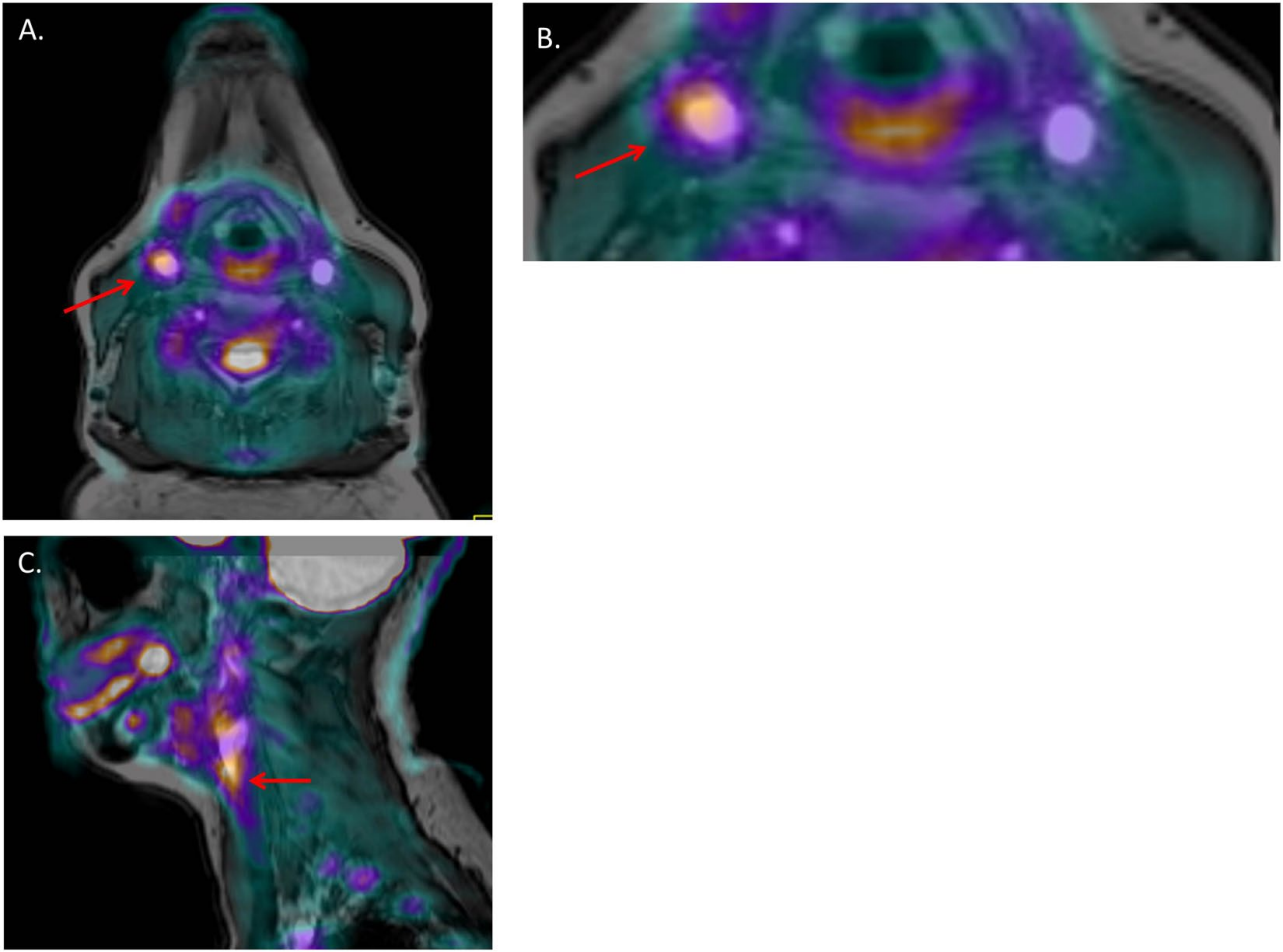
Provides an example of a carotid FDG-PET-MRI in an RA patient. (A) A cross sectional image at the level of the plaque. (B) Provides a more magnified image of the plaque on this slice which demonstrates increased uptake around the vessel wall where plaque has been identified (highlighted by the red arrow). (C) A coronal section in the same patient with the red arrow highlighting the area of increased uptake within the plaque in this view.
